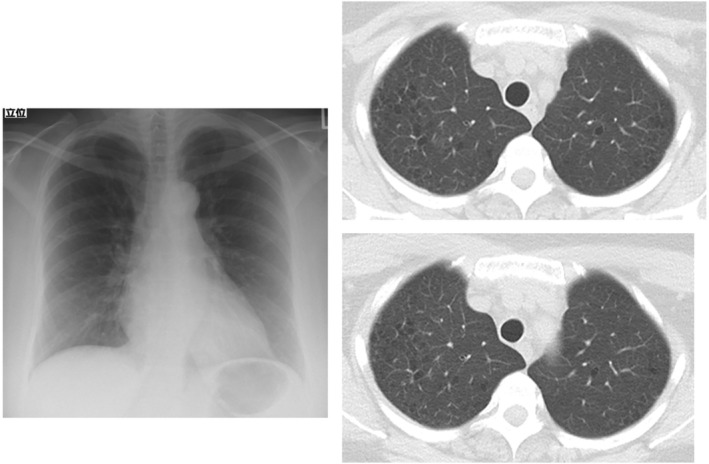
Baseline chest computed tomography showing mild centrilobular emphysematous change.
